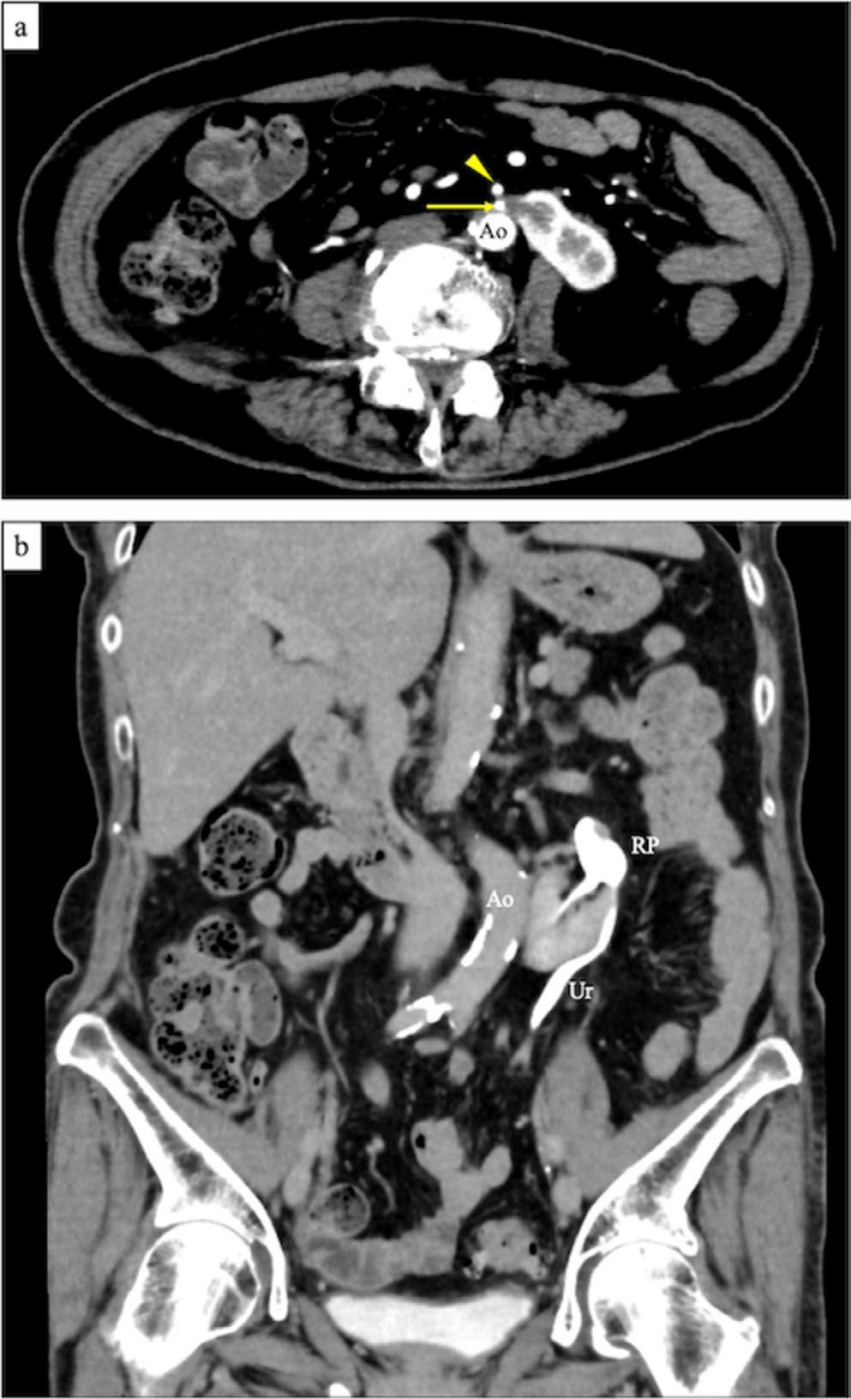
Contrast‐enhanced computed tomography at 3 months postoperatively. (a) The isthmus was resected posterior to the inferior mesenteric artery (arrowhead). The laparoscopic stapler line is indicated by the arrow. (b) Contrast enhancement of the renal pelvis of the left kidney and the left ureter. No evidence of urinoma or residual right renal pelvis/calyces is observed at the remaining isthmus.
